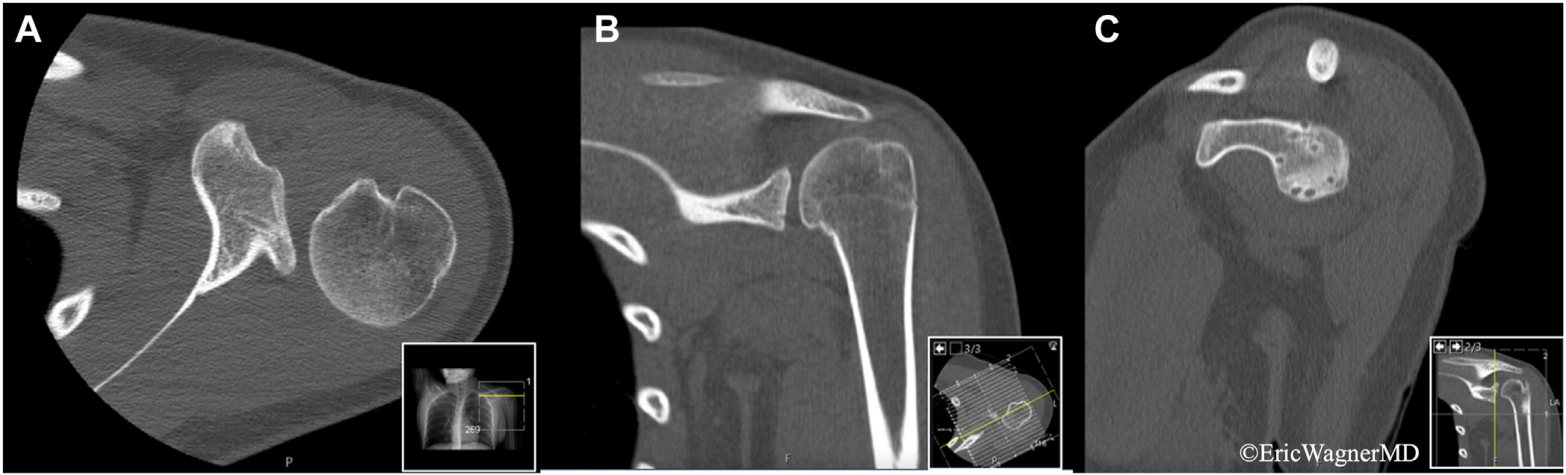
Computed tomography (CT) of left shoulder in axial (A), coronal (B), and sagittal (C) planes. Axial view demonstrates posterior subluxation of shoulder. Sagittal view demonstrates multiple glenoid cortical defects representing postsurgical anchor tracks from previous capsulolabral fixation.
